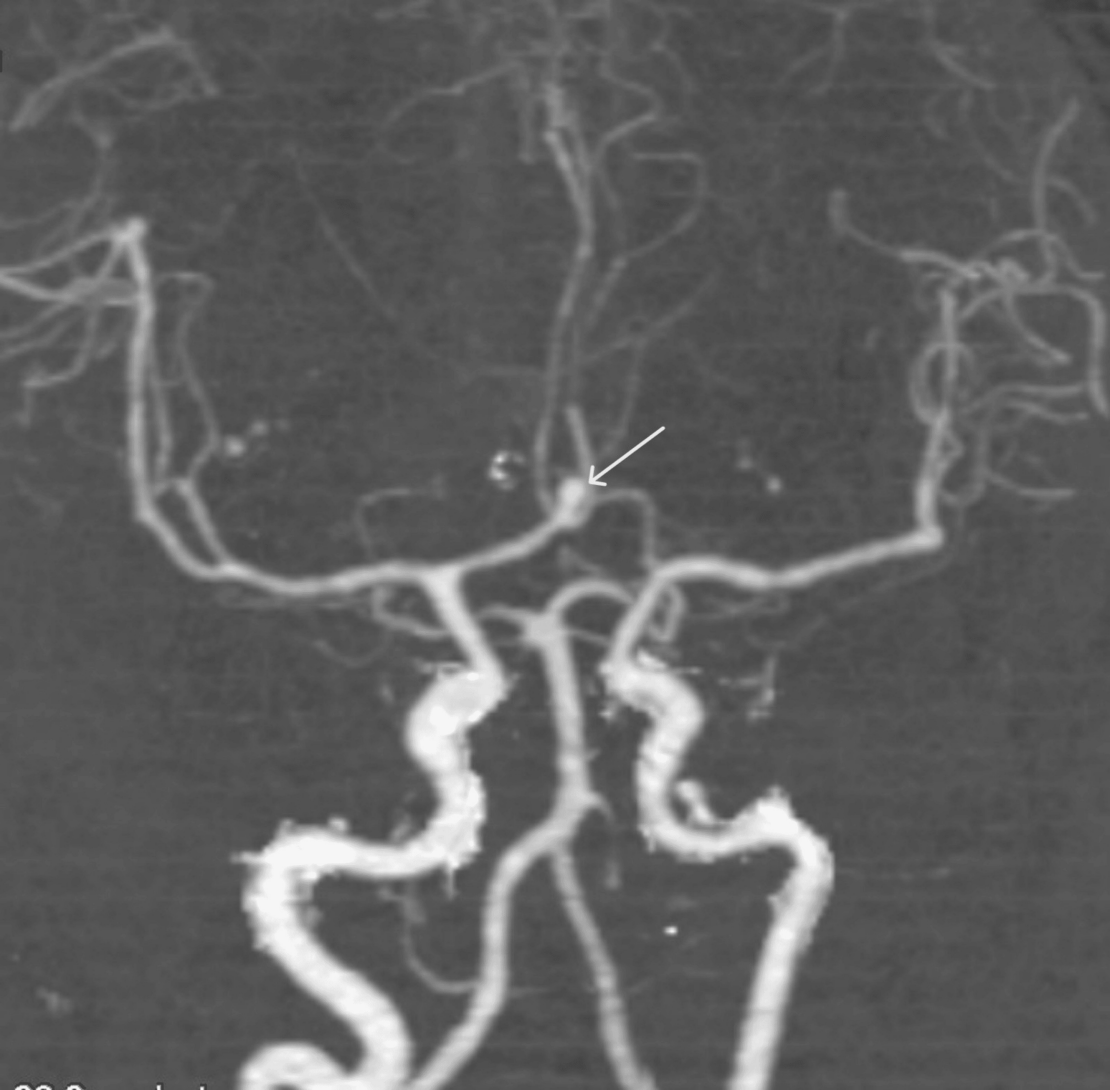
Axial MIP CT angiography image showing a saccular aneurysm at the ACOM (white arrow) MIP: maximum intensity projection, CT: computed tomography, ACOM: anterior communicating artery
